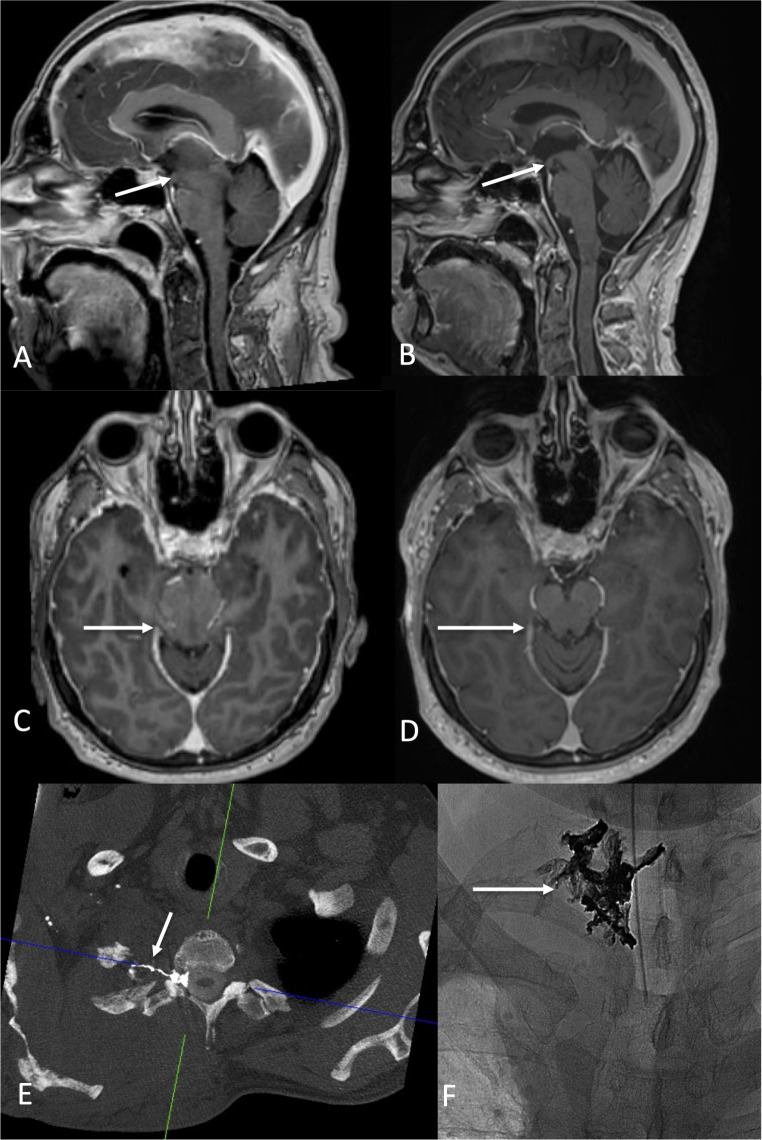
A 64-year-old man presented with disinhibition, dizziness and logorhoea so that he was not able to work as a teacher any longer. MRI shows severe midbrain sagging with an extremely low mamillo-pontine distance (A: arrow) and an elongated midbrain (B: arrow). Dynamic CT myelography discloses a CSF venous fistula at the right-sided T2 nerve root (C: arrow). Following transvenous embolization (F: arrow points to the Onyx cast), MRI changes are regredient (D, E: arrows) and the patient reported on marked improvement
